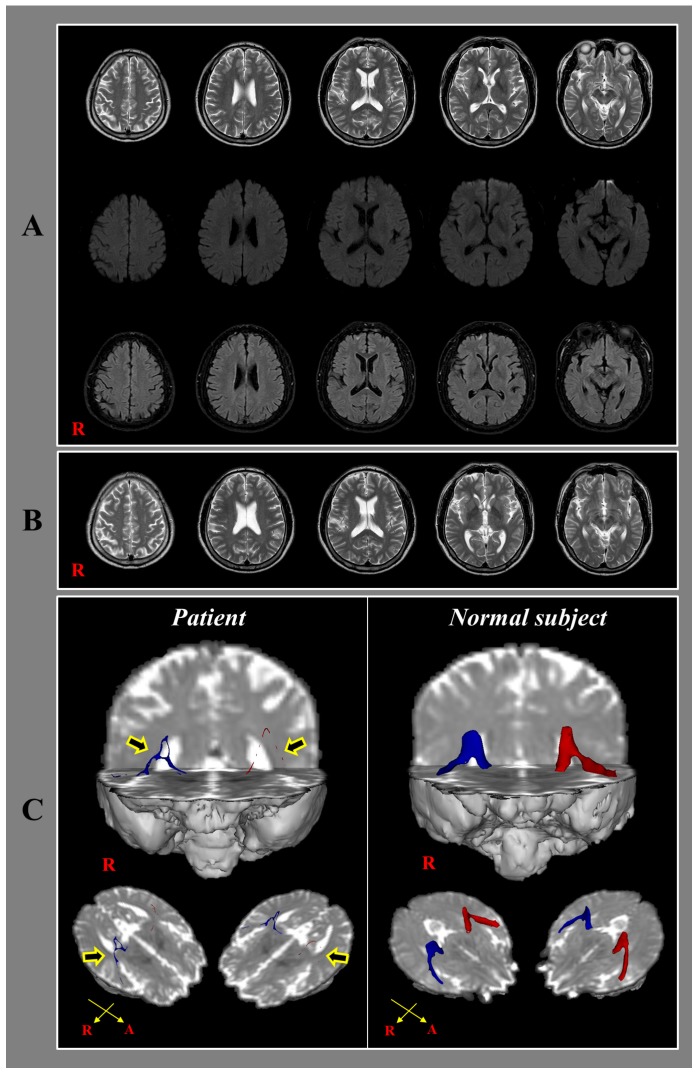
(A) T2-weighted (upper row), diffusion weighted (middle row) and fluid-attenuated inversion recovery images (lower row) taken 2 weeks after onset reveals no abnormal lesion; (B) T2-weighted images taken 2.5 years after onset shows no abnormal lesion; (C) 2.5-year diffusion tensor tractography for the auditory radiation in the patient shows severe narrowing and tearing (arrows) in both hemispheres compared with a normal subject (47-year old female).
